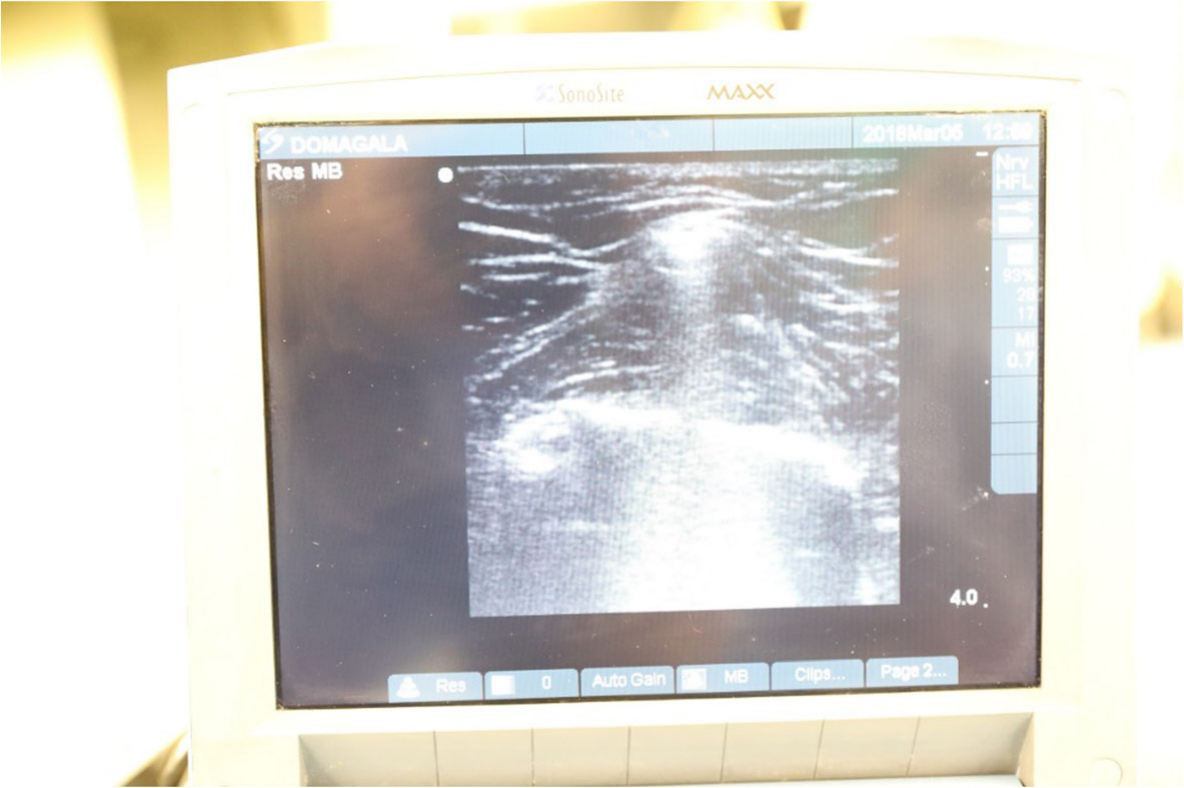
Ultrasound image demonstrating placement of the guide along femoral shaft and bone
